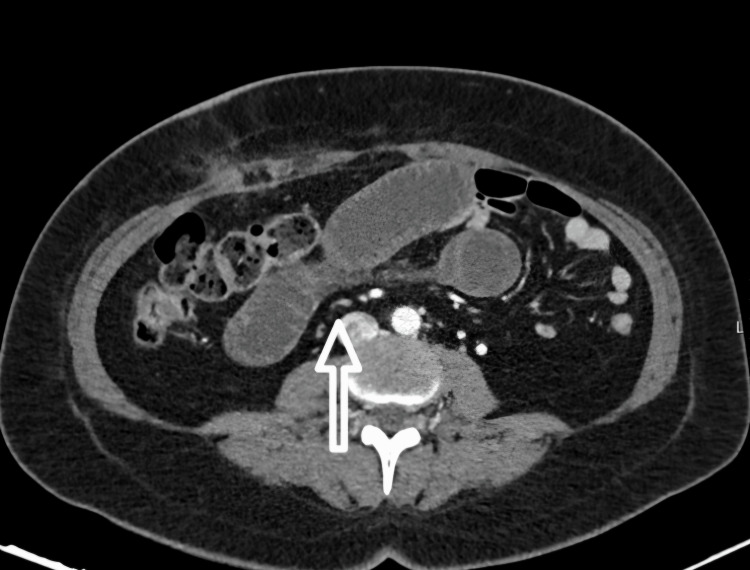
Case 3 - Axial CT scan showing dilated small bowel loop with transition zone Contrast-enhanced axial CT image revealing a markedly distended proximal small bowel loop (highlighted by the white arrow), with a subtle transition zone posterior to the superior mesenteric vessels. These findings were suggestive of mechanical obstruction and prompted urgent laparoscopic exploration, which confirmed Petersen’s hernia with early reversible ischemia.
